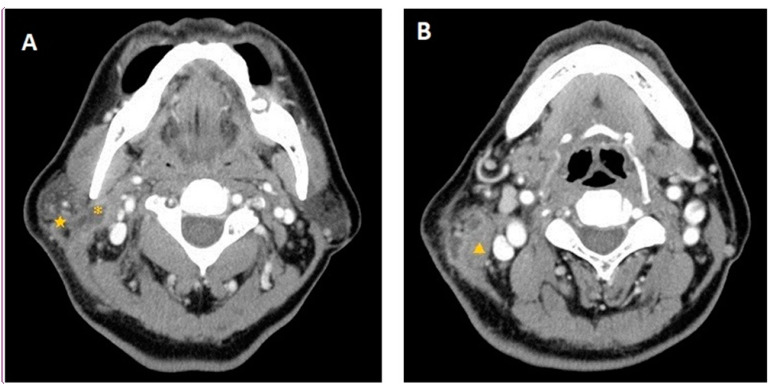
Case 2: Computed tomography images of the neck show (A) focal fluid accumulation in the right parapharyngeal (*) and right parotid (★) spaces; and (B) right sternocleidomastoid muscle swelling (▲).
